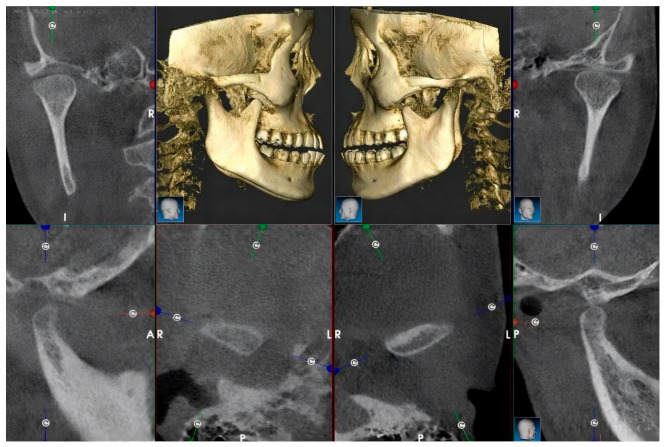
Sagittal CBCT images demonstrating standardized measurement of TMJ joint spaces.
